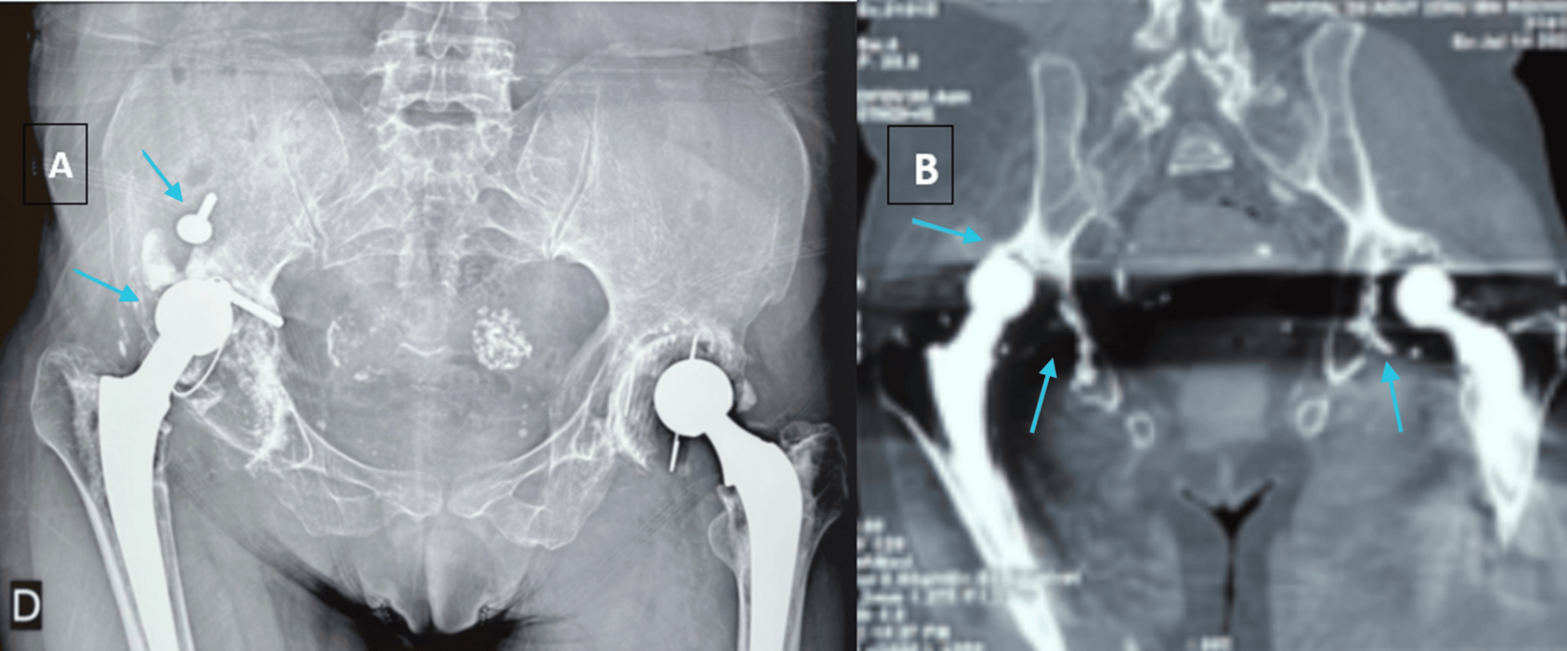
Pelvic radiograph (A) and coronal CT scan (B) demonstrating loosening of the right total hip prosthesis. A. Anteroposterior pelvic radiograph showing bilateral total hip prostheses. The right prosthesis demonstrates clear signs of loosening, with superior migration of the femoral component and fracture of one of the fixation screws, associated with peri-prosthetic bone changes. B. Coronal CT reconstruction showing mechanical loosening of the right prosthesis, with marked peri-acetabular bone loss and displacement of the femoral component.
